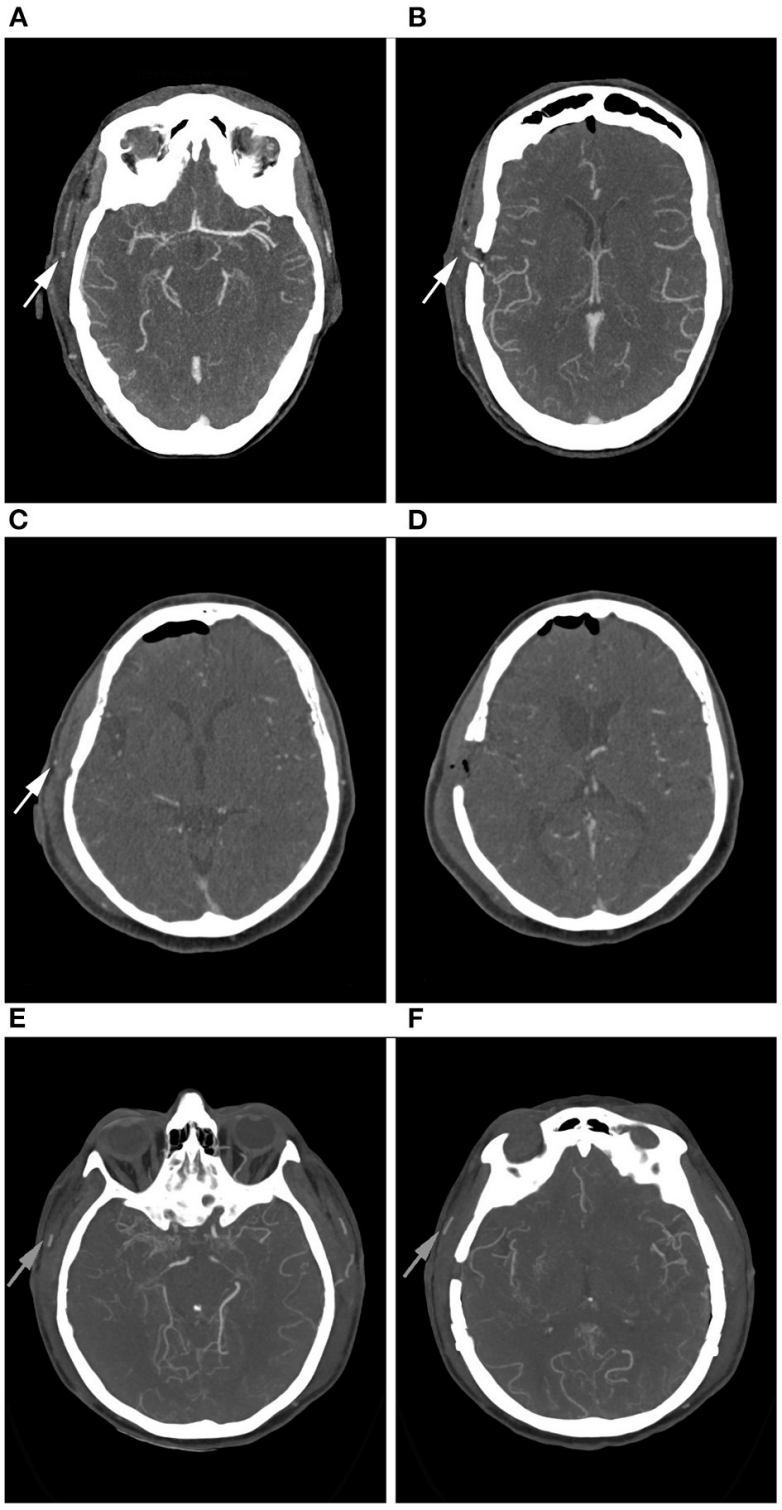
Example of right-sided STA-MCA bypasses in CTA. (A,B): Bypass is patent. The proximal (A) and the distal (B) parietal branch of the STA are visible (arrows). (C,D): Bypass of uncertain patency. Only the proximal branch of the STA is visible (C, arrow), the distal branch is not clearly contrasted (D). (E,F): Bypass appears occluded. Neither the proximal (E) nor the distal (F) parietal branch of the STA are contrasted. Good contrast enhancement of the frontal branch of the STA (arrows).
